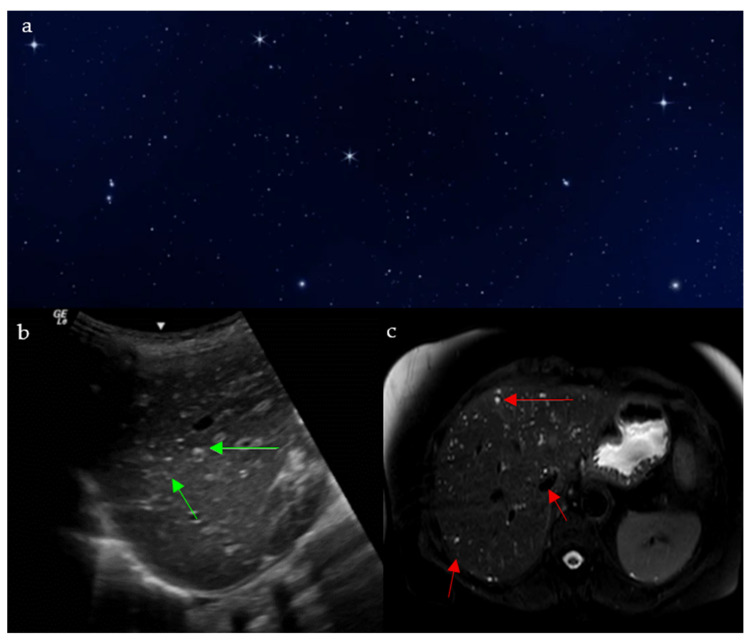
The appearance of a starry sky (a), resembling bright echogenic dots throughout a background of decreased liver parenchymal echogenicity on ultrasound (green arrows) (b), and small innumerable T2 hyperintense bile duct hamartomas and biliary microhamartomas, scattered throughout the T2 hypointense hepatic parenchyma (red arrows) (c).
